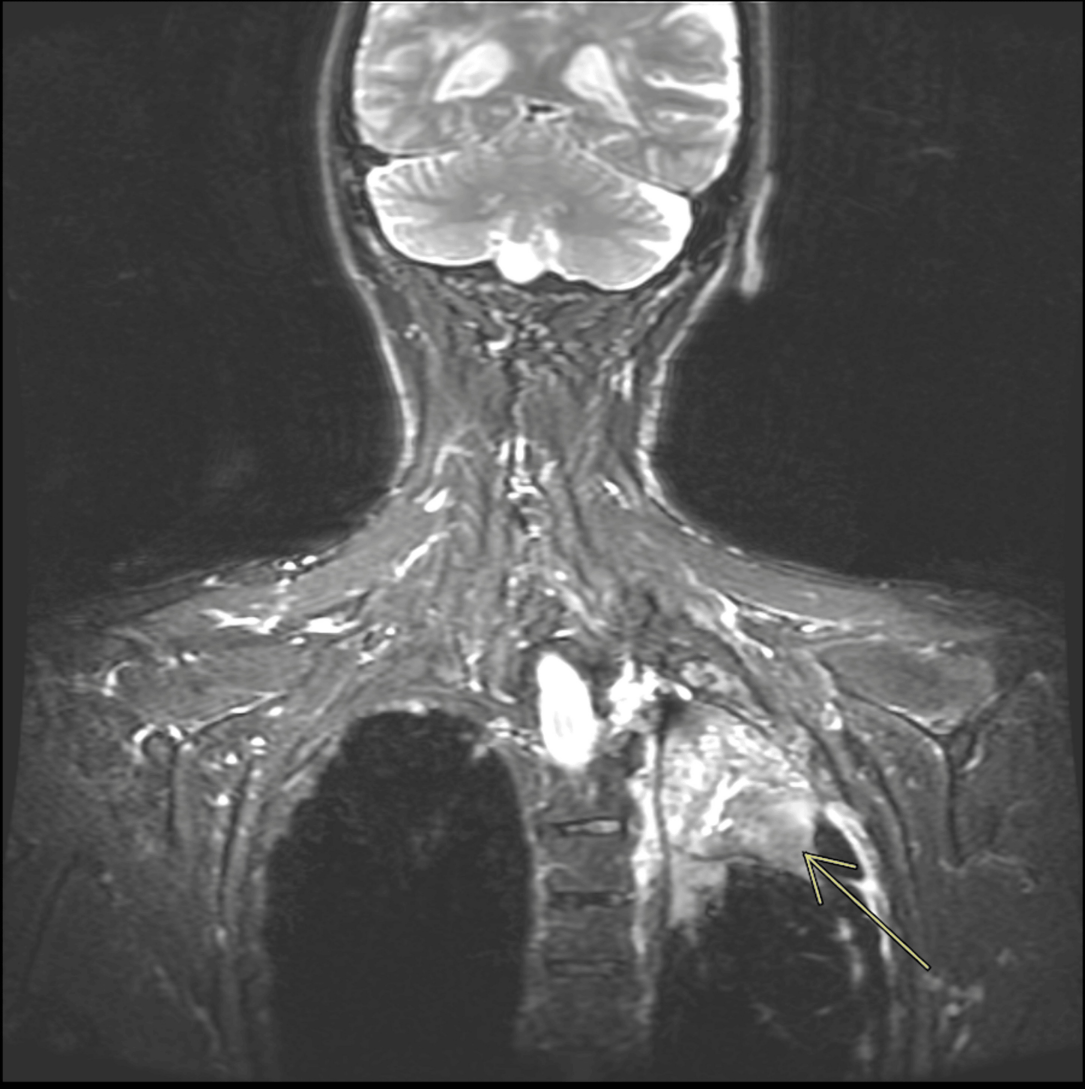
Coronal T2 MRI sequence with fat suppression of the brachial plexus showing possible impingement of the left recurrent laryngeal nerve. No definite evidence of brachial plexus involvement The arrow points to a heterogeneously enhancing soft tissue mass at the left lung apex measuring 57 x 87 x 87 mm
